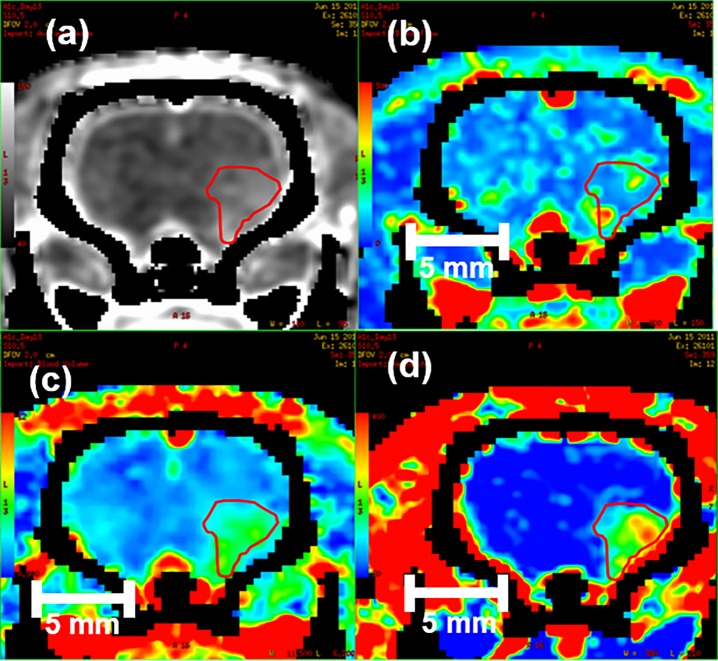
Delineation of tumor and CT perfusion data. (a) Tumor. (b) Tumor blood flow (BF). (c) Tumor blood volume (BV). (d) Tumor permeability-surface area (PS) product.
